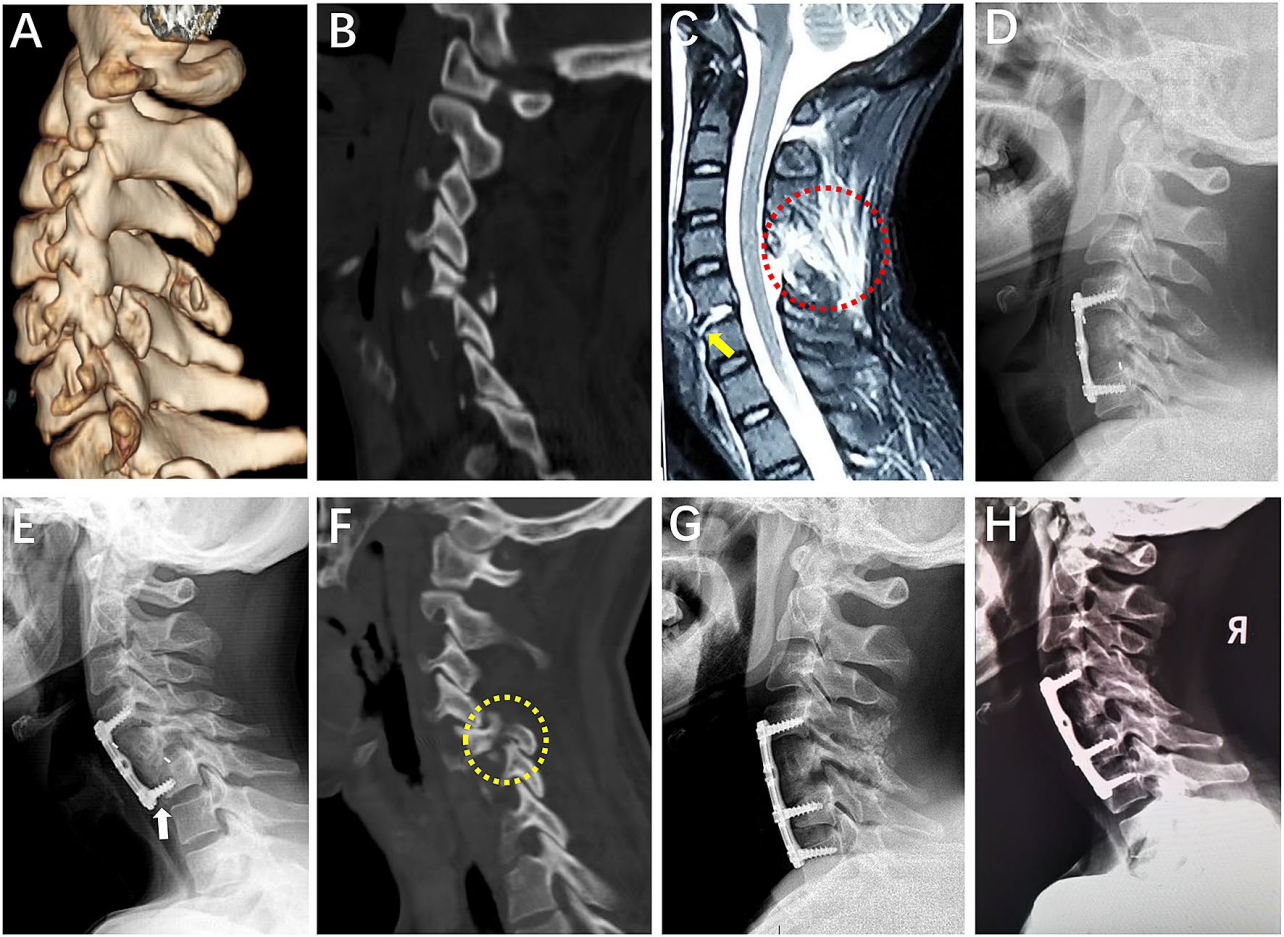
A 40-year-old man who was diagnosed as type IIB injury pattern of UCLF. Left side facet interlocking with ipsilateral inferior articular process fracture at C5-6 motion segment was discovered on CT three-dimensional reconstruction appearance (A, B). Sagittal T2-weighted MR image showed that the patient had a rupture of anterior longitudinal ligament and intervertebral disc (yellow arrow), and severe posterior ligament complex injury (red circle) (C). The patients had a successful closed reduction by preoperative skull traction, and anterior C5 corpectomy and fusion was performed (D). At 4 months follow-up after surgery, the patient had a sever neck pain and dysphagia due to failed reduction with migration of screws (E) and facet dislocation (yellow circle) at the injury segment (F). A revision surgery was performed by posterior facet release, reduction and autogenous bone fusion combined with extending the anterior fusion segment (G). The cervical spinal alignment was stable and the graft fusion was satisfactory at 20 months follow-up after revision surgery (H).
